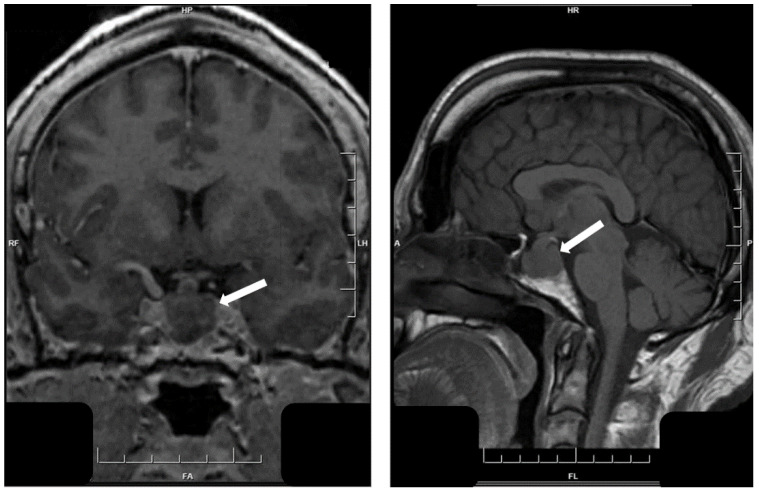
Magnetic resonance imaging of immature PIT1-lineage tumor. This large tumor invades laterally into both cavernous sinuses (arrow, left) extends upwards, forming a “snowman”-like mass herniating above the diaphragma sella (arrow, right).
